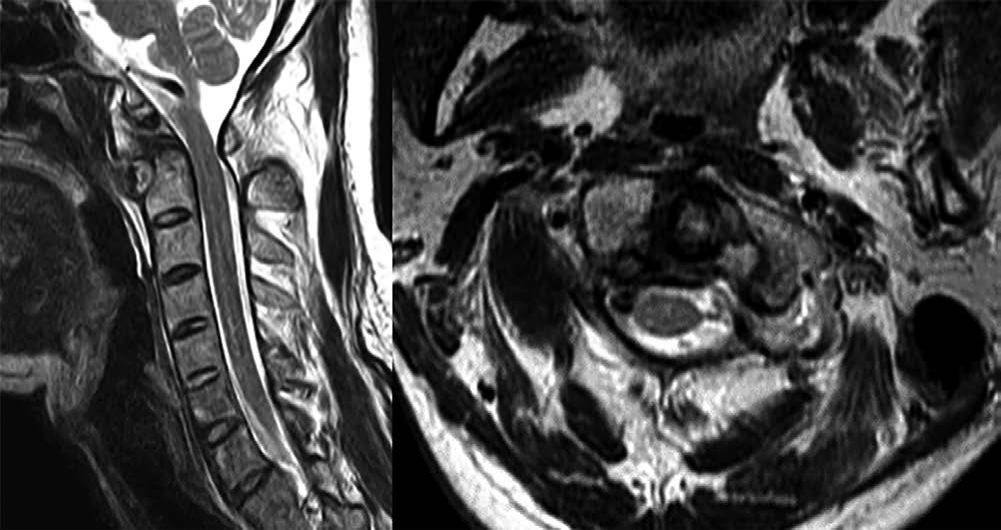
Preoperative cervical magnetic resonance image without contrast enhancement. Although the space available for the spinal cord was significantly reduced, there was no evidence that the spinal cord was compressed or injured.
